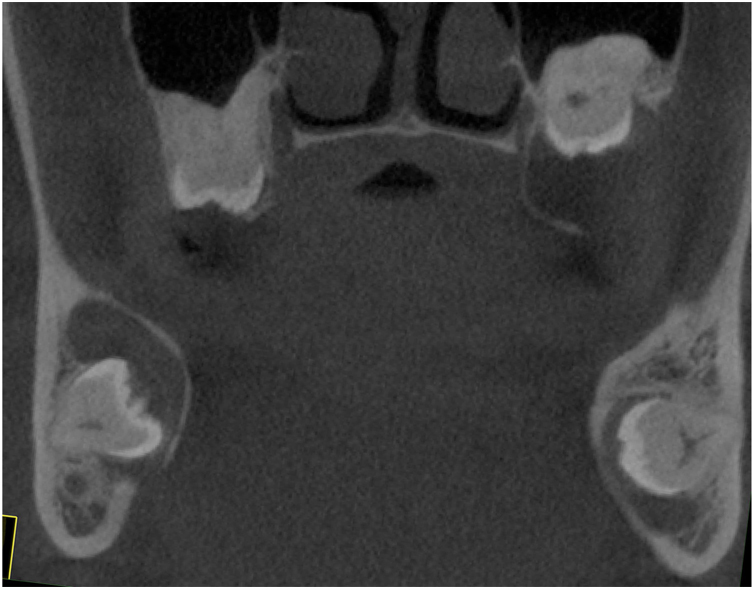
Coronal CBCT image showing expansive cystic lesion surrounding the crowns of impacted molars in maxilla and mandible.
